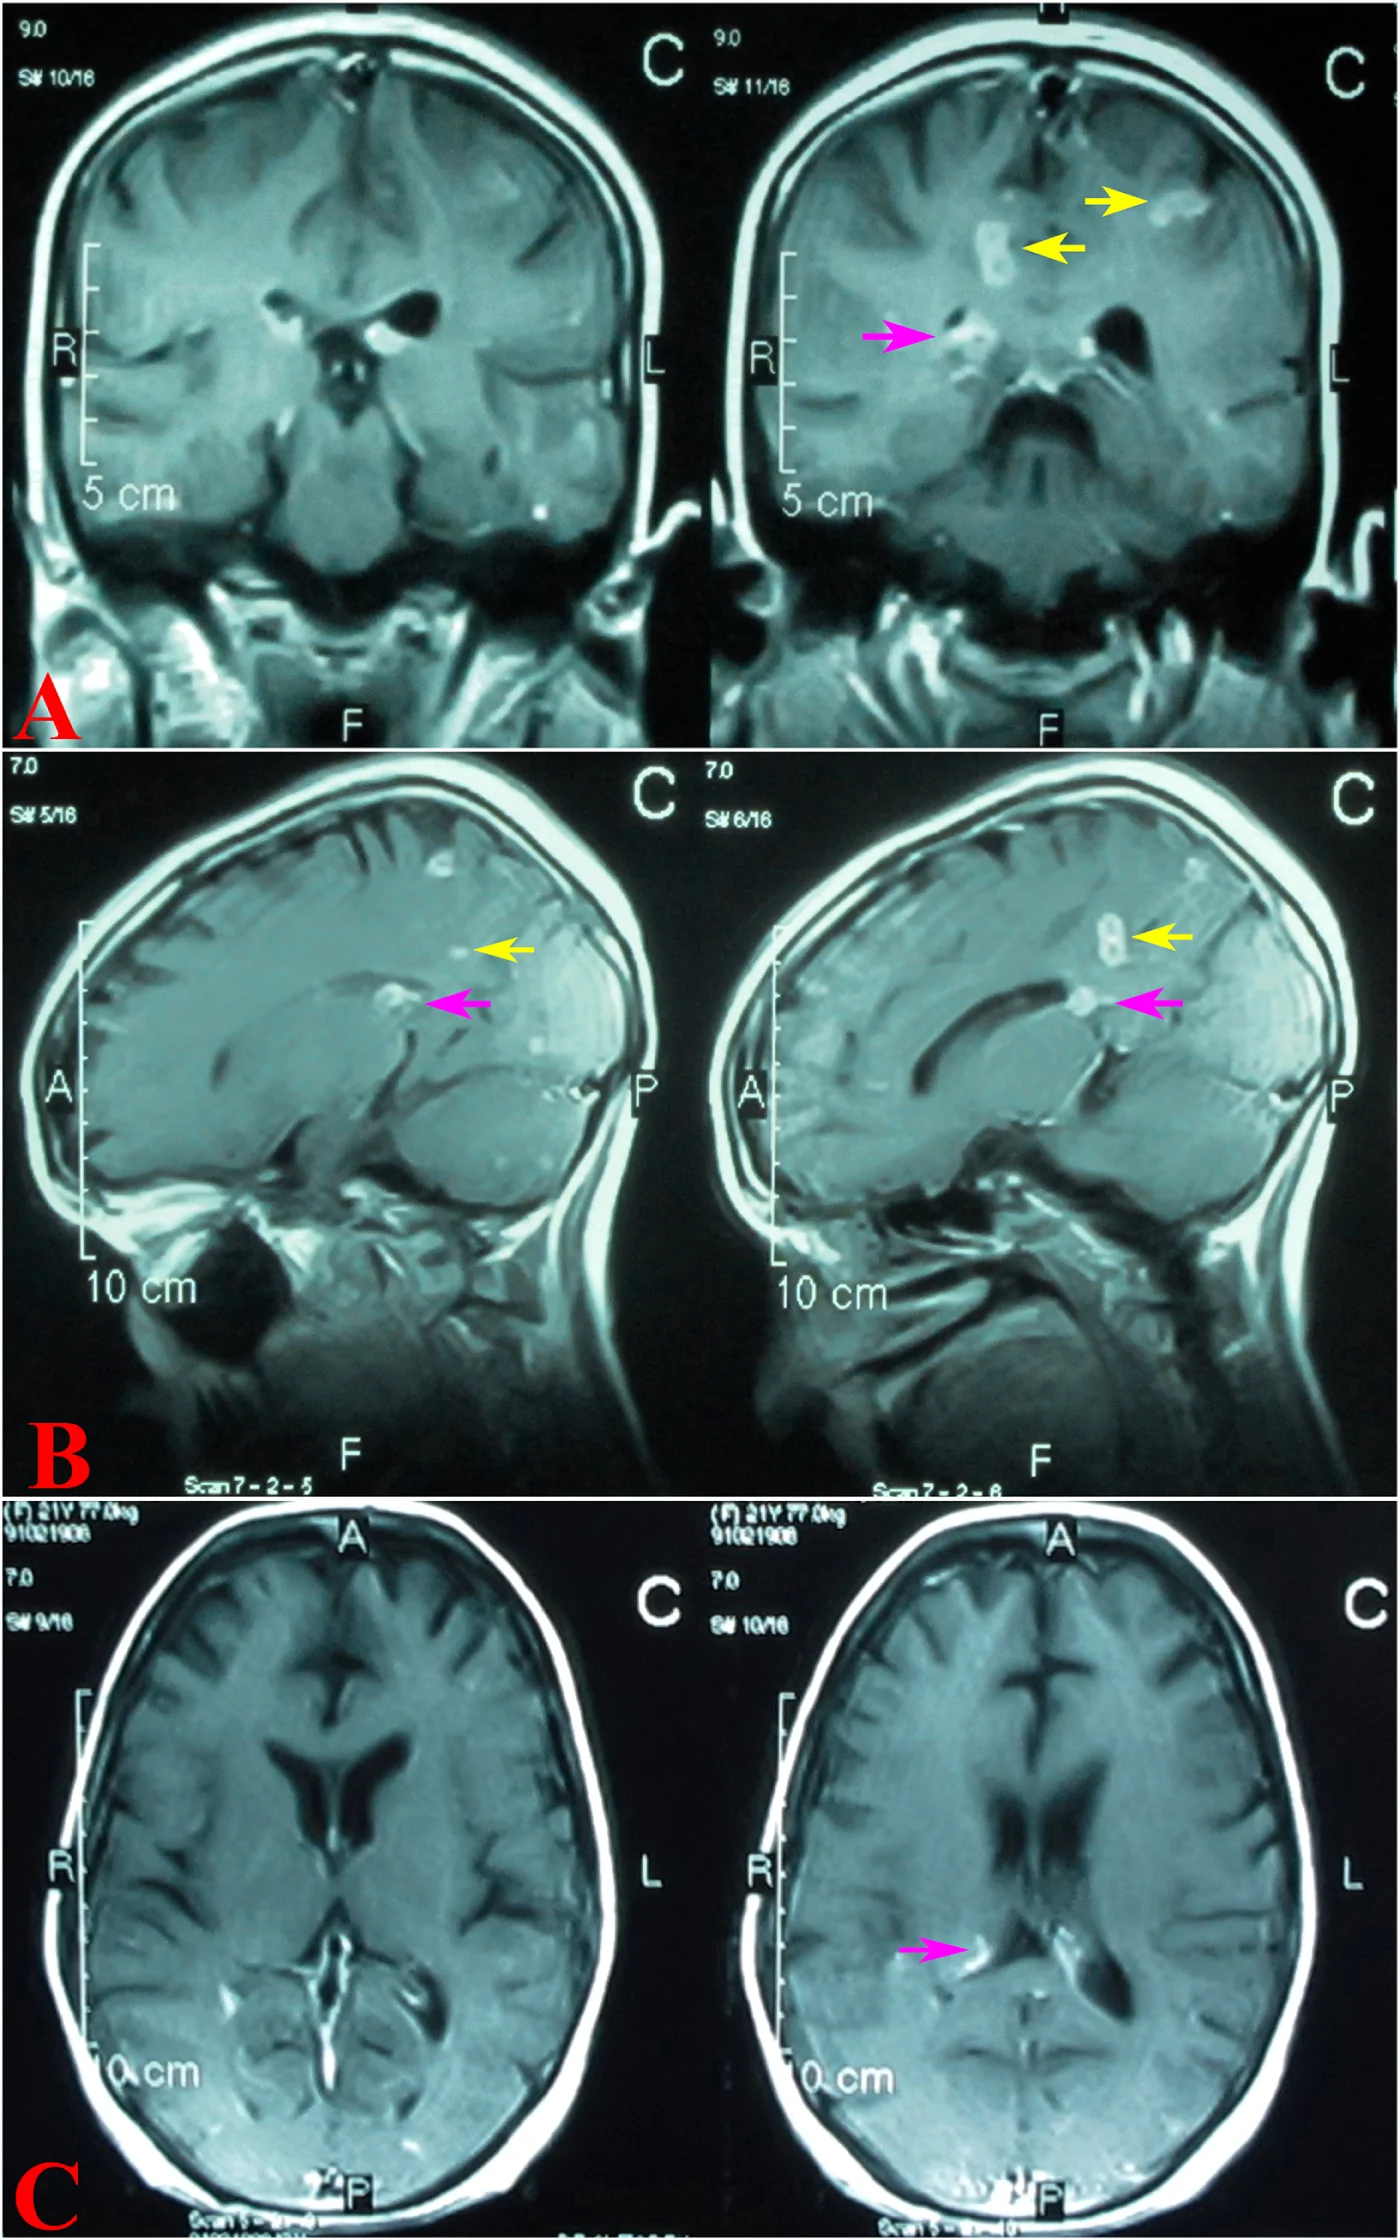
Brain MRI 1 year after treatment revealed the disappearance of many intracranial tuberculomas (yellow arrows), as well as partial recovery of ventriculitis (magenta arrows) in T1-weighted MRI: coronal (A), sagittal (B), and horizontal (C) views.
